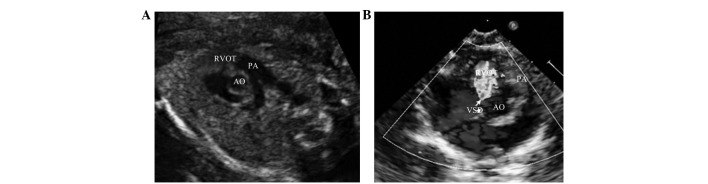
Comparison of a heart diagnosed as normal in a 23-week fetus by echocardiography and as having a ventricular septal defect (membranous part) in a 1-month infant by echocardiography recheck. (A) Fetal echocardiography indicating a normal aorta short axis view. (B) Infant recheck echocardiography revealing a ventricular level shunting signal of the membranous ventricular septum in the color Doppler aorta short axis view. AO, aorta; RVOT, right ventricle of outflow tract; PA, pulmonary artery; VSD, ventricular septal defect.
